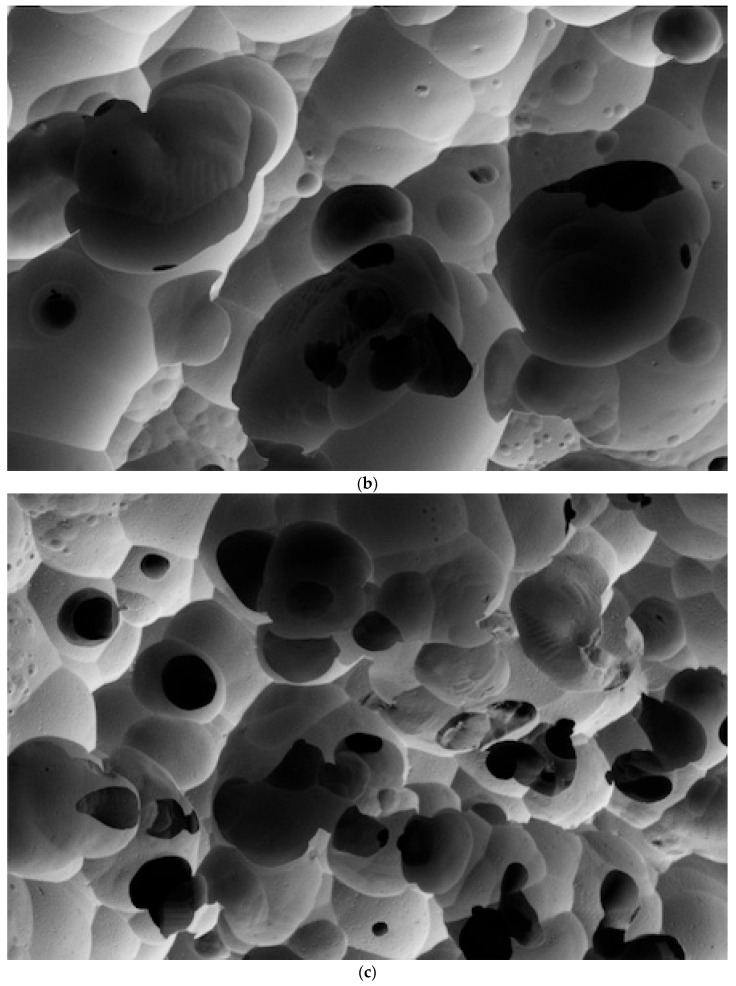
(a) The RelyX Veneer Try-in Paste cleaned with the Rinse and Dry method di-played a completely clean surface with no residual paste (Score: None). (b) The RelyX Veneer Try-in Paste cleaned with Rinse and Dry followed by an Ultrasonic Bath in Distilled Water showed a completely clean surface with no residual paste (Score: None). (c) The RelyX Veneer Try-in Paste cleaned with Rinse and Dry followed by an Ultrasonic Bath in Alcohol also showed a completely clean surface with no residual paste (Score: None).
